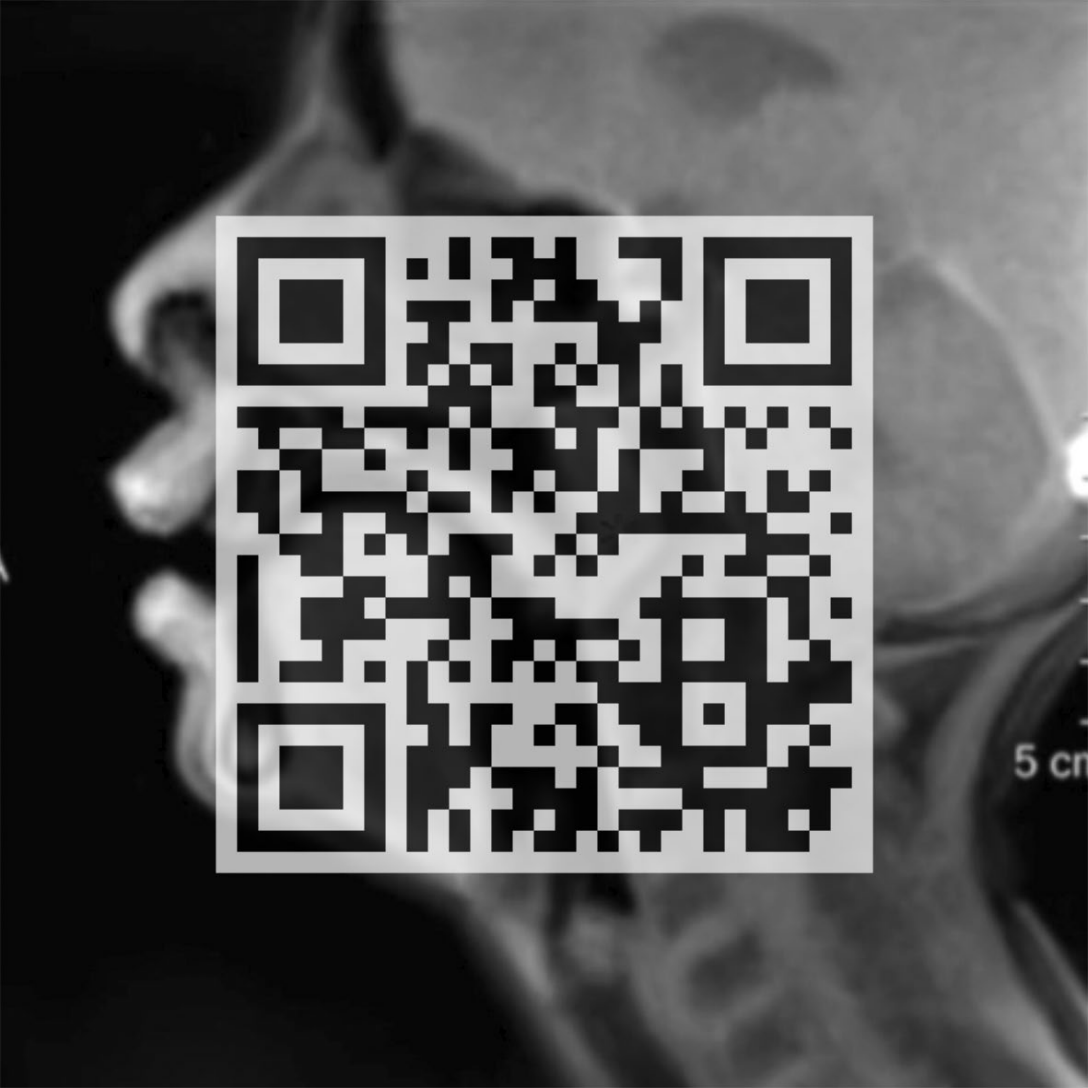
Video 9 Real-time MRI (proton density weighted) of the pharynx of a 5-year-old girl after surgery for a mucous cleft palate. While speaking the word “crocodile,” closure of the nasopharynx cannot be observed. The soft palate was surgically fixed to the posterior pharyngeal wall (red asterisk) and is therefore permanently attached here [4]
